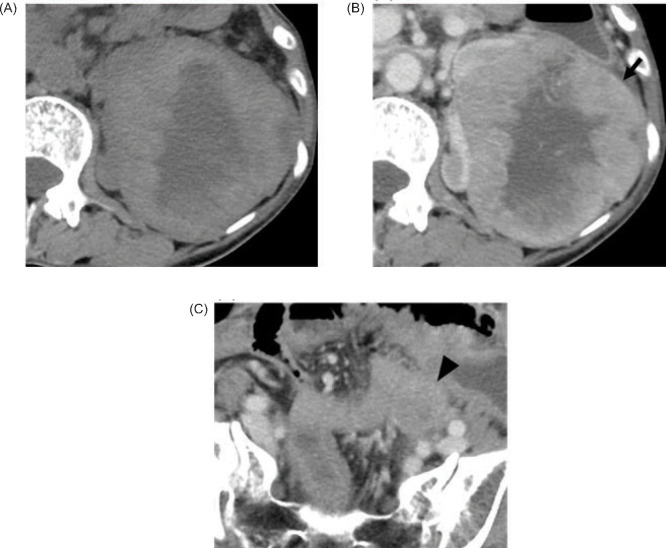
At non-enhanced CT (A), the margins of the mass on the left kidney were hyperattenuating compared with the renal parenchyma, and the interior portion of the mass was hypoattenuating. At contrast-enhanced CT (B, C), the solid portions enhanced less intensely compared with the adjacent renal cortex and enhanced more intensely compared with the adjacent renal medulla. The interior portion of the mass was poorly contrasted, and necrosis was suspected. A capsule-like structure was observed at the tumour margins (arrow). There was lymphadenopathy approximately 25 × 25 mm in the left common iliac area (arrowhead), and metastasis is suspected.
